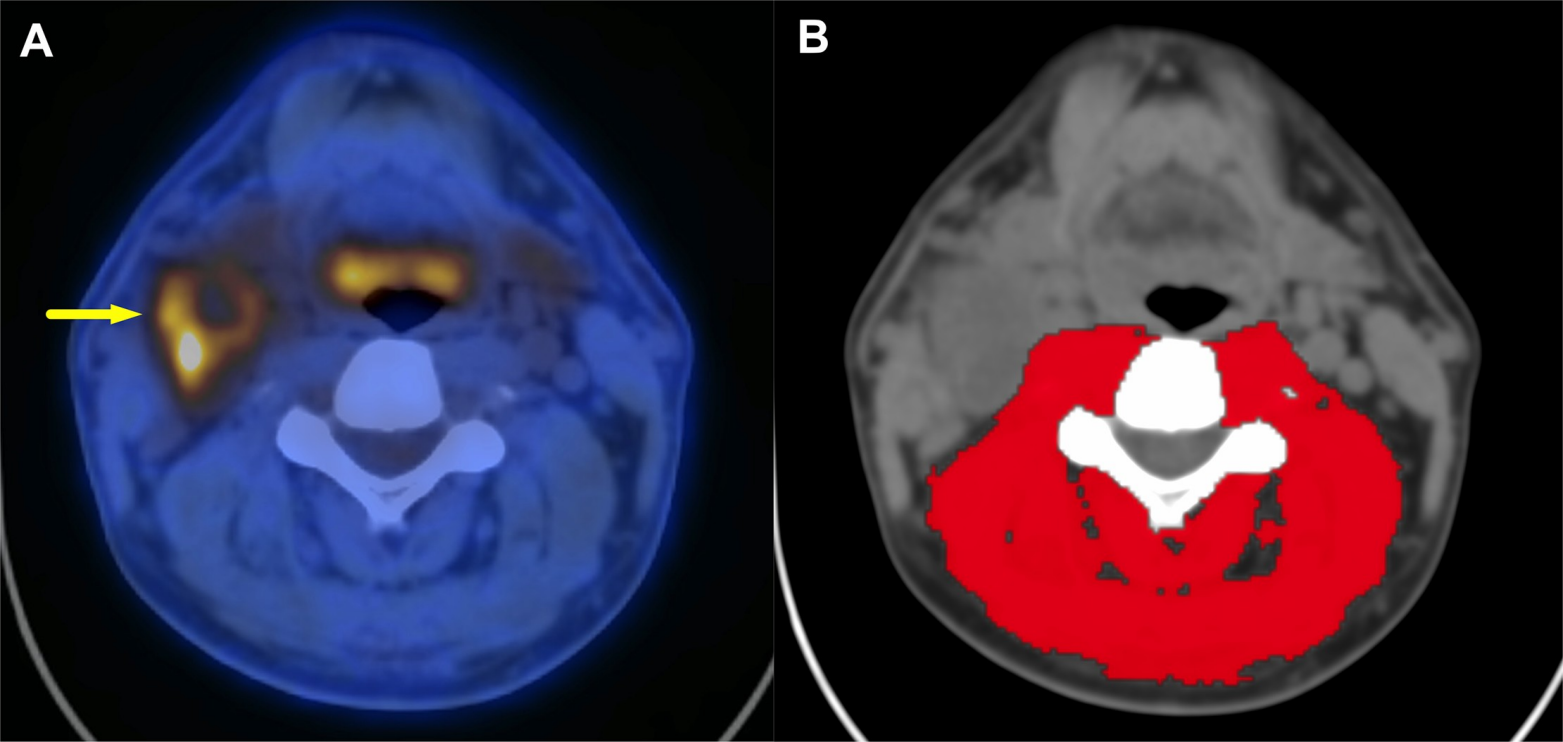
Representative fusion PET/CT (A) and CT (B) images used to measure the paravertebral muscle area at C3. A 57-year-old male patient with right tonsillar cancer. Metastatic lymphadenopathy is detected at right cervical levels II–III with invasion of the right sternocleidomastoid muscle.
